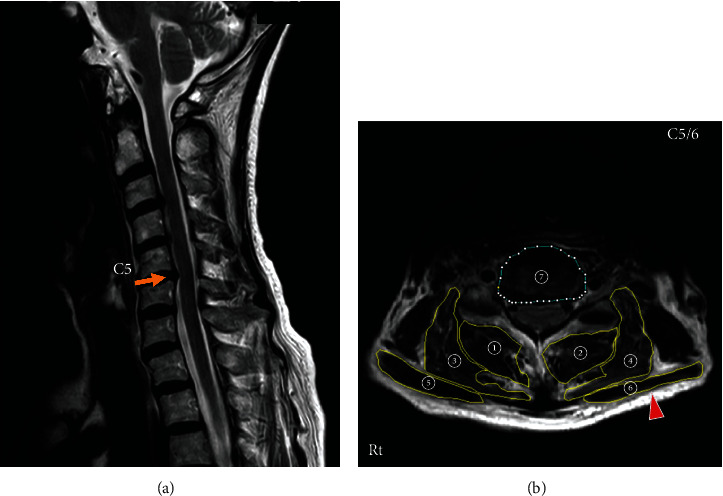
T2-weighted magnetic resonance (MR) sagittal image (a) and axial image at C5-C6 (b) of the patient's cervical spine at the first visit. (a) Cervical alignment was slightly kyphotic, and the C5-C6 intervertebral disc protruded posteriorly (arrow). (b) The cervical extensor muscles were atrophic. The trapezius muscles showed a stretched morphology (arrowhead). ➀➁: deep layers of the cervical extensor muscles (semispinalis cervicis and multifidus). ➂➃: lateral-dorsal layers of the cervical extensor muscles (splenius capitis, splenius cervicis, and longissimus). ➄➅: trapezius. ➆: C5-C6 disc.
